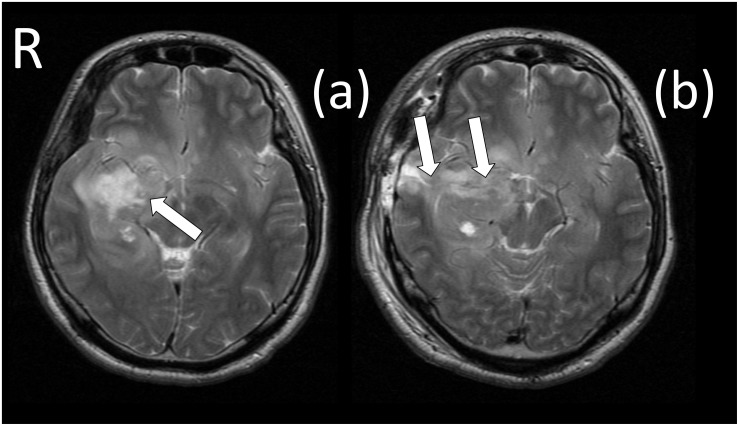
Magnetic resonance imaging (MRI) scans before and after surgery. (a) The white arrow identifies the brain tumor (meningioma), which was located in the right temporal region. (b) The white arrows identify the surgically induced scars that reached to the amygdala.
